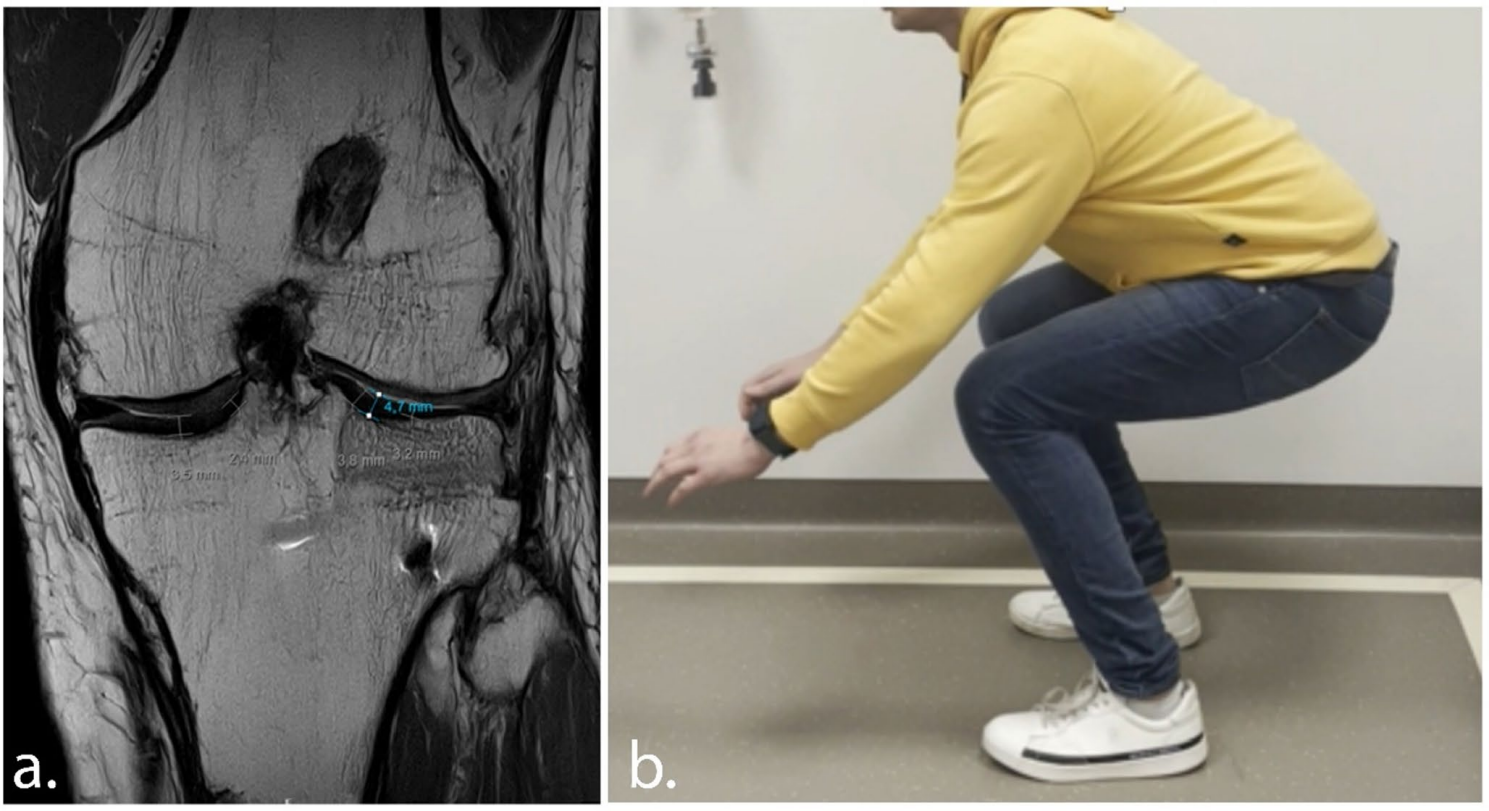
At one-year follow-up, the patient showed favourable clinical recovery, with coronal proton density–weighted turbo spin echo (PD-TSE) MRI of the lateral tibial plateau allograft demonstrating good integration and preservation of cartilage (a) and good squatting ability (b)
